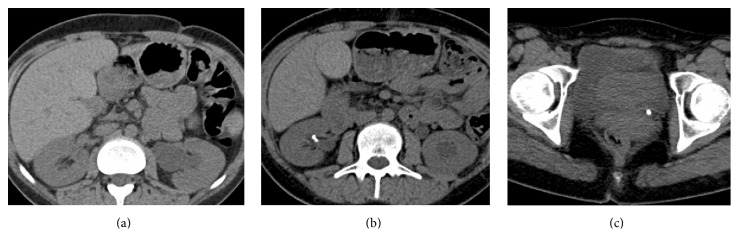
(a–c) Axial CT images of a 21-year-old female with left renal angle pain on a background of cystic fibrosis. (a) Noncontrast axial CT at the level of the renal hila demonstrates left hydronephrosis. The absence of normal pancreatic tissue and increased attenuation of the liver possibly due to haemosiderin deposition are also evident. (b) Axial CT image below the renal hila demonstrating a nonobstructing calculus in the right renal pelvis and left hydroureter. (c) Axial CT image at the level of the bladder demonstrating an obstructing left lower ureteric calculus.
